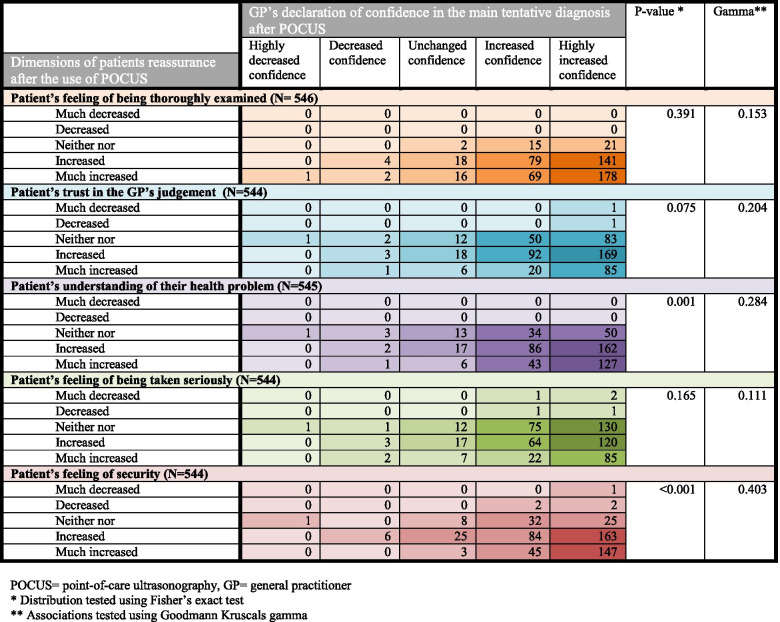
Associations between patient reassurance and general practitioners’ confidence in diagnosis. POCUS = point-of-care ultrasonography, GP = general practitioner. * Distribution tested using Fisher’s exact test.  Associations tested using Goodmann Kruscals gamma
